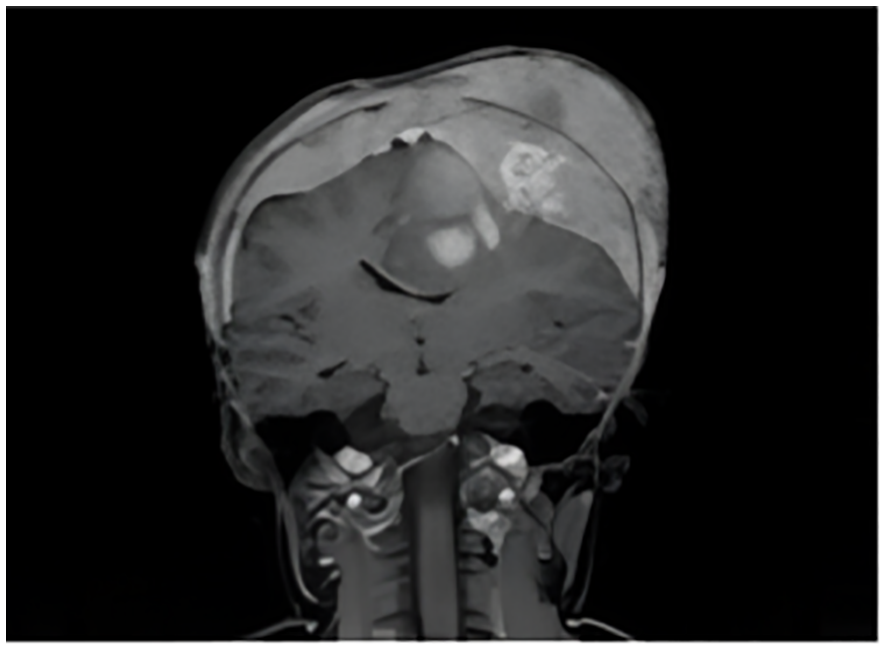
Contrast-enhanced whole-body MRI showed an expansive and infiltrative lesion in the left parietal region.
